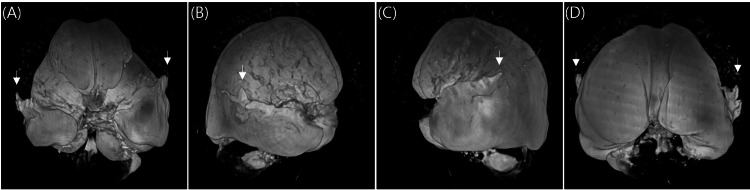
Three-dimensional reconstruction of T2-weighted MRI images of the ventricle. (A) Anterior view. (B) Lateral (right) view. (C) Lateral (left) view. (D) Posterior view. White arrows indicate ventricular ruptures.
